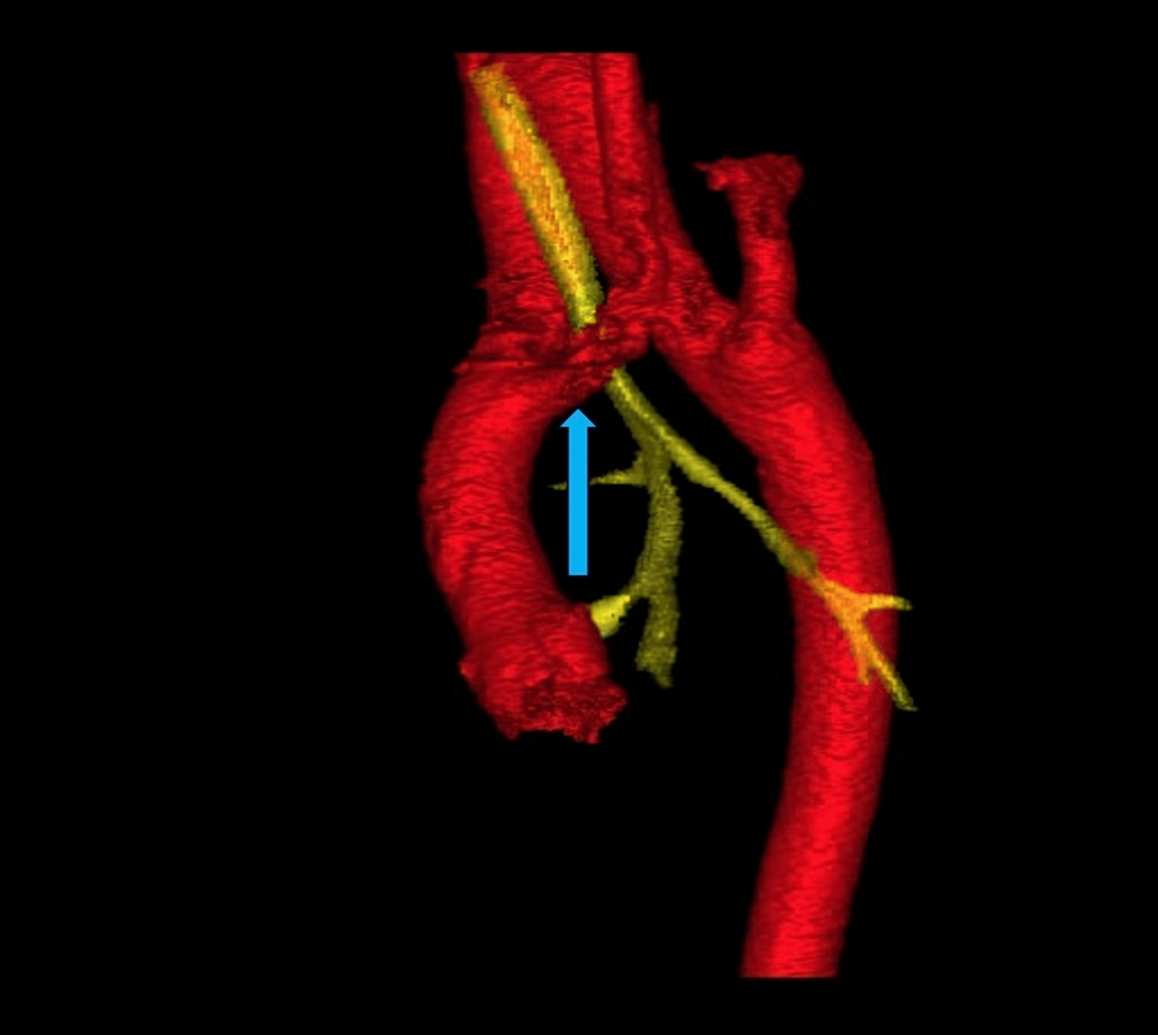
Volume-rendered 3D model from chest CTA demonstrating the VR (red) compressing the trachea (yellow) in the posterior view The blue arrow highlights the ASA forming the VR. ASA, aberrant subclavian artery; CTA, CT angiography; VR, vascular ring
